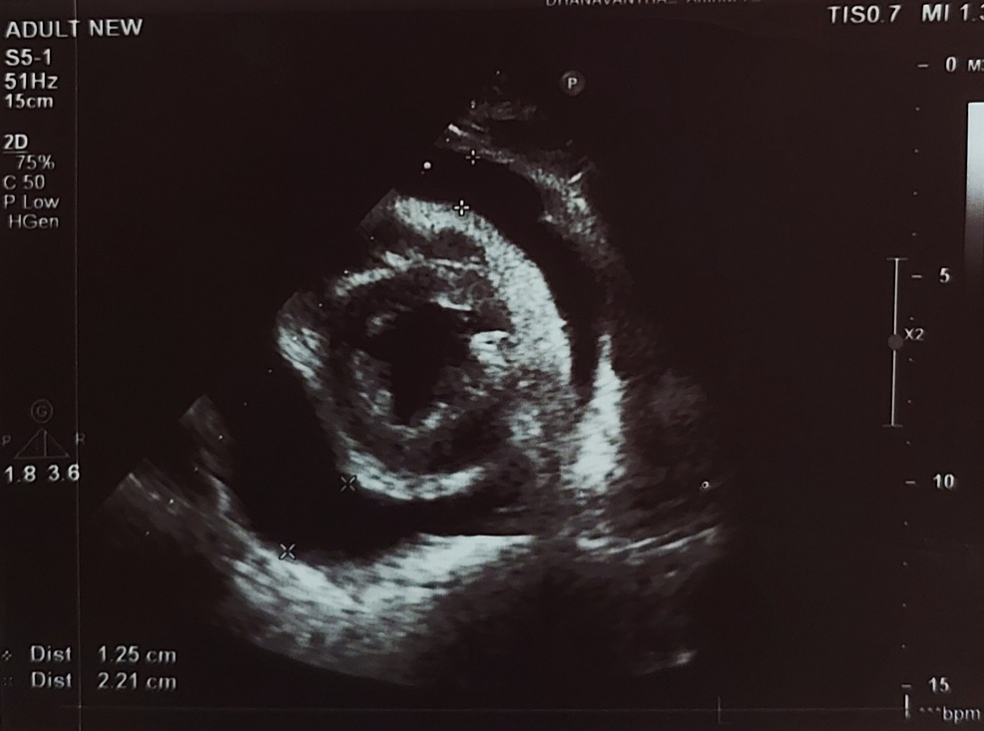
2D echocardiogram shows pericardial effusion
